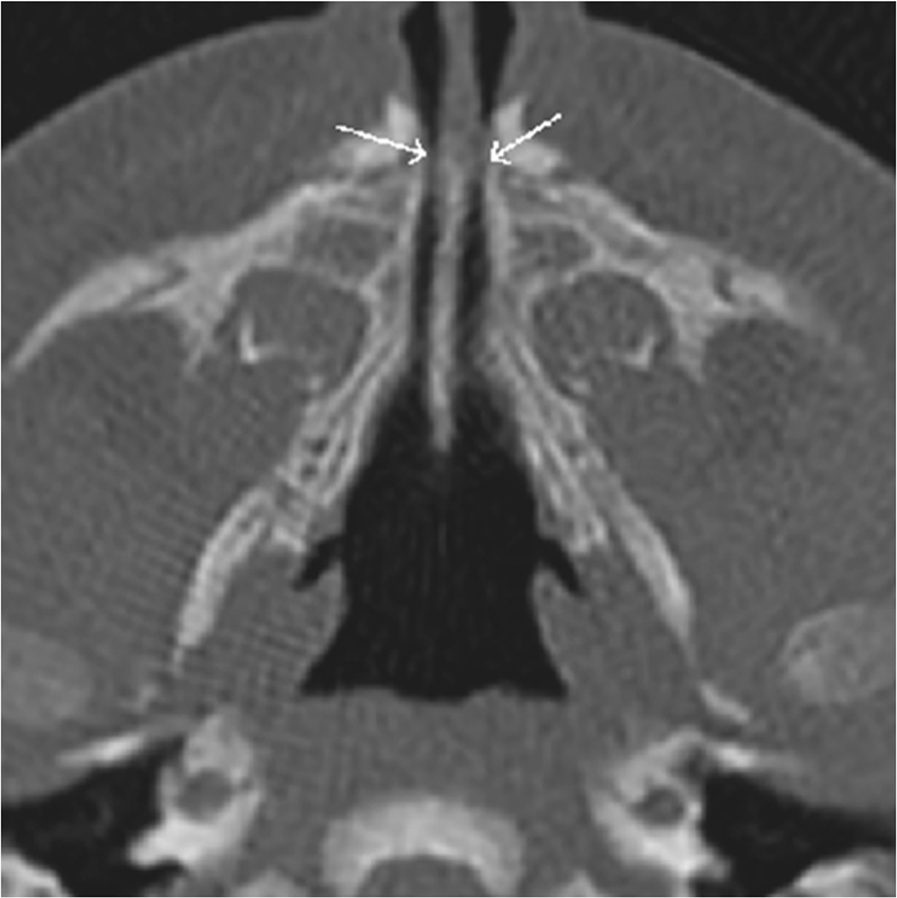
Pre-operative CT scan showing the nasal pyriform aperture stenosis.
